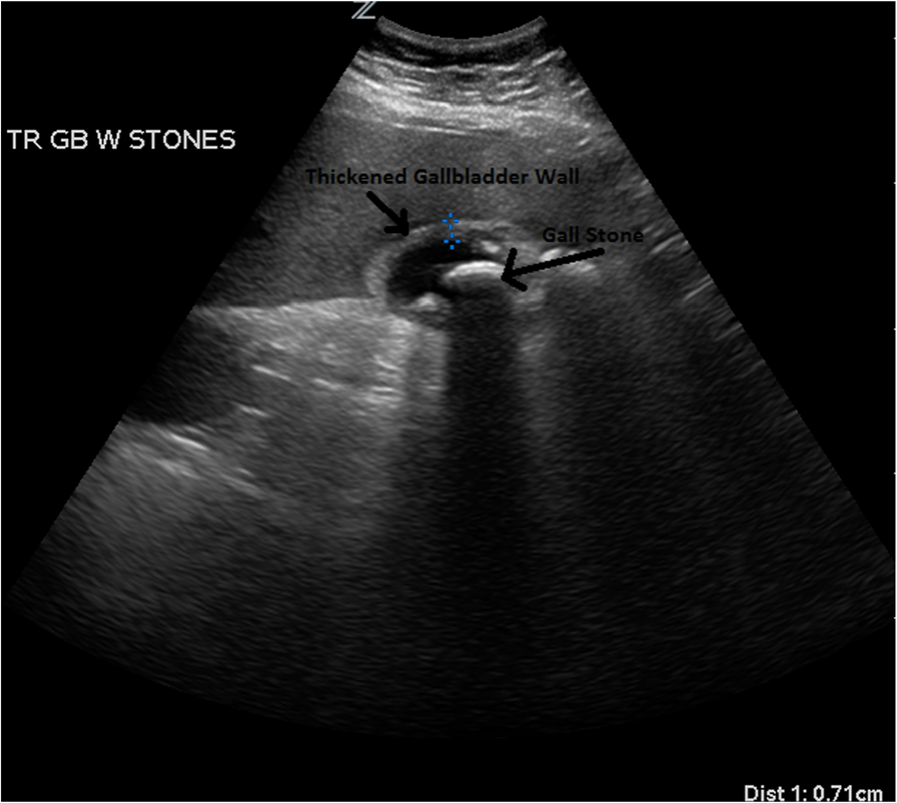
Ultrasound of a patient with acute cholecystitis. A very large gallstone with significant surrounding edema can be seen.
